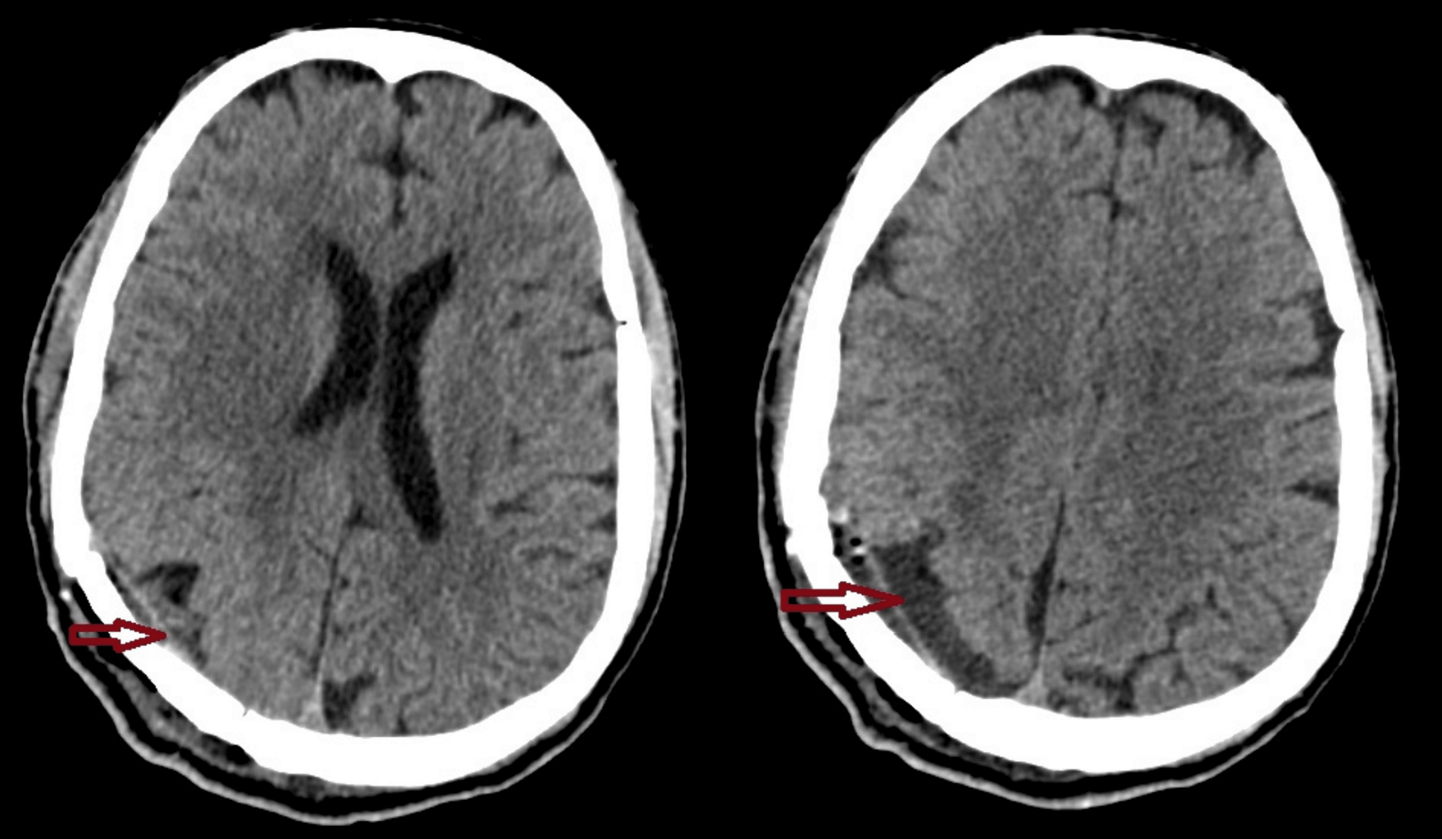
A postoperative CT scan confirmed complete lesion resection
